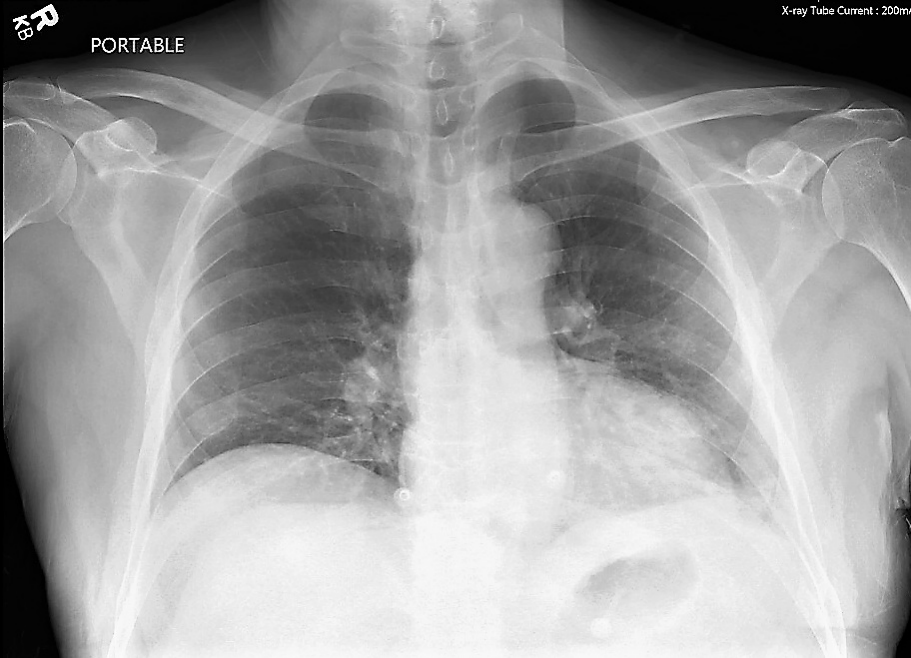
Anterior-posterior chest radiograph showing no acute cardiopulmonary process
